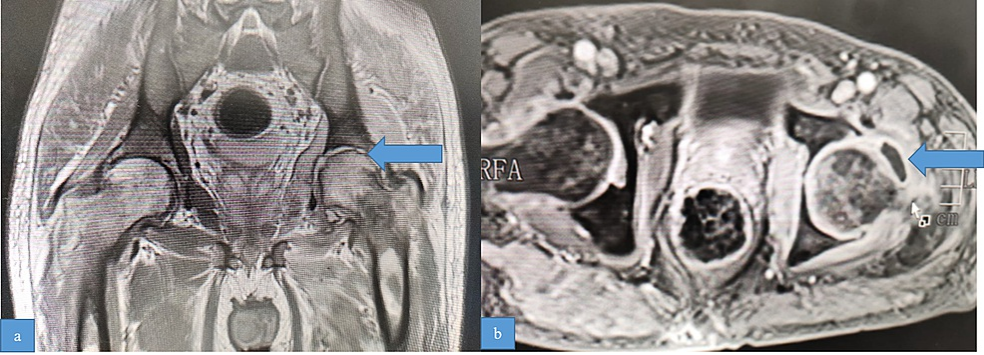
Post-contrast T1 FS sagittal and T1-weighted coronal images showing edema in the head and neck of the left femur, cortical irregularity of the head with loss of articular cartilage, and small joint effusion. FS: fat suppression; T1: time constant
